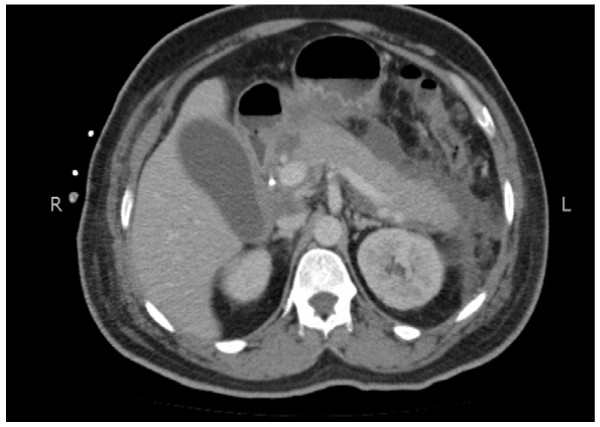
Axial view of the computed tomography scan of our patient showing soft tissue thickening at the gall bladder neck and proximal bile duct region.
